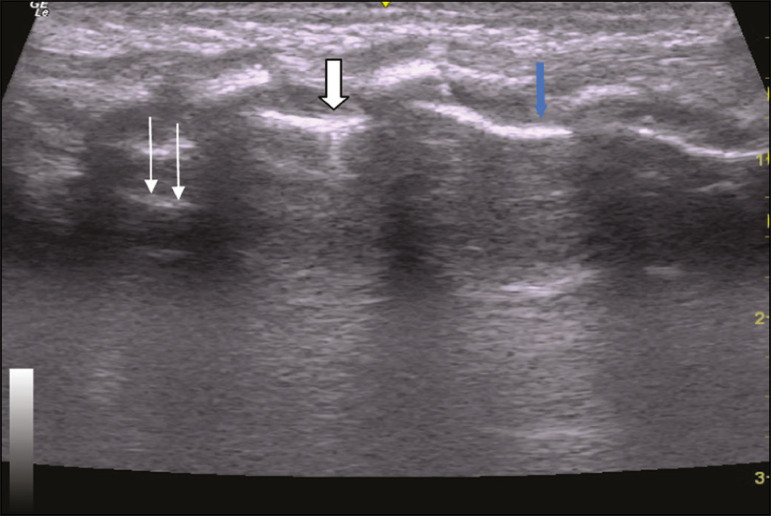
Asymptomatic COVID-19-negative neonate with normal ultrasound examination results. Presence of A lines (thin arrows), few B lines (filled arrow), regular pleural line (blue arrow).
